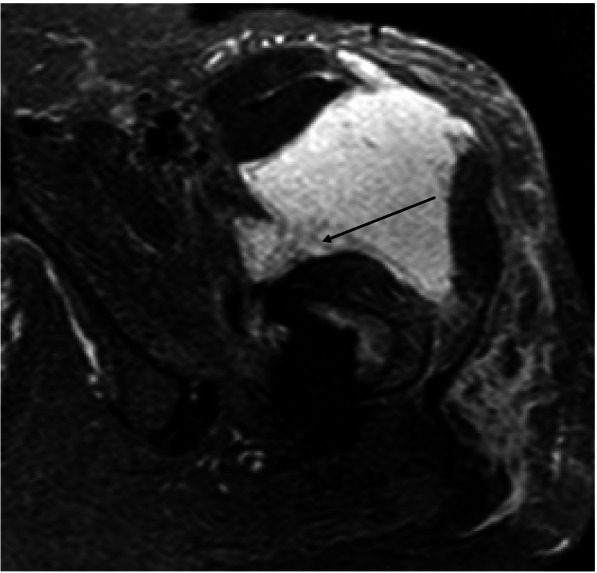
On axial MARS-STIR-MRI layering (black arrow) is detectable in the fluid accumulation
